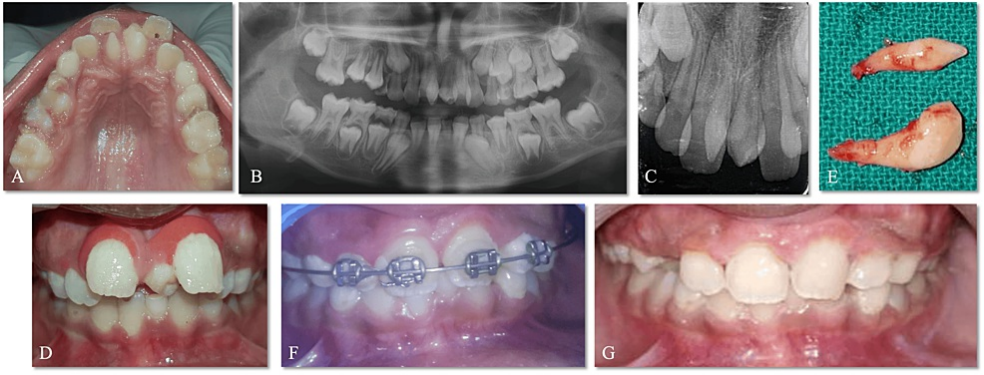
Case 5 A: pre-operative maxillary occlusal view; B: pre-operative orthopantomogram (OPG); C: pre-operative intraoral periapical radiograph (IOPA); D: pre-operative frontal view; E: extracted mesiodentes; F: two-by-four appliance therapy; G: post-operative frontal view
